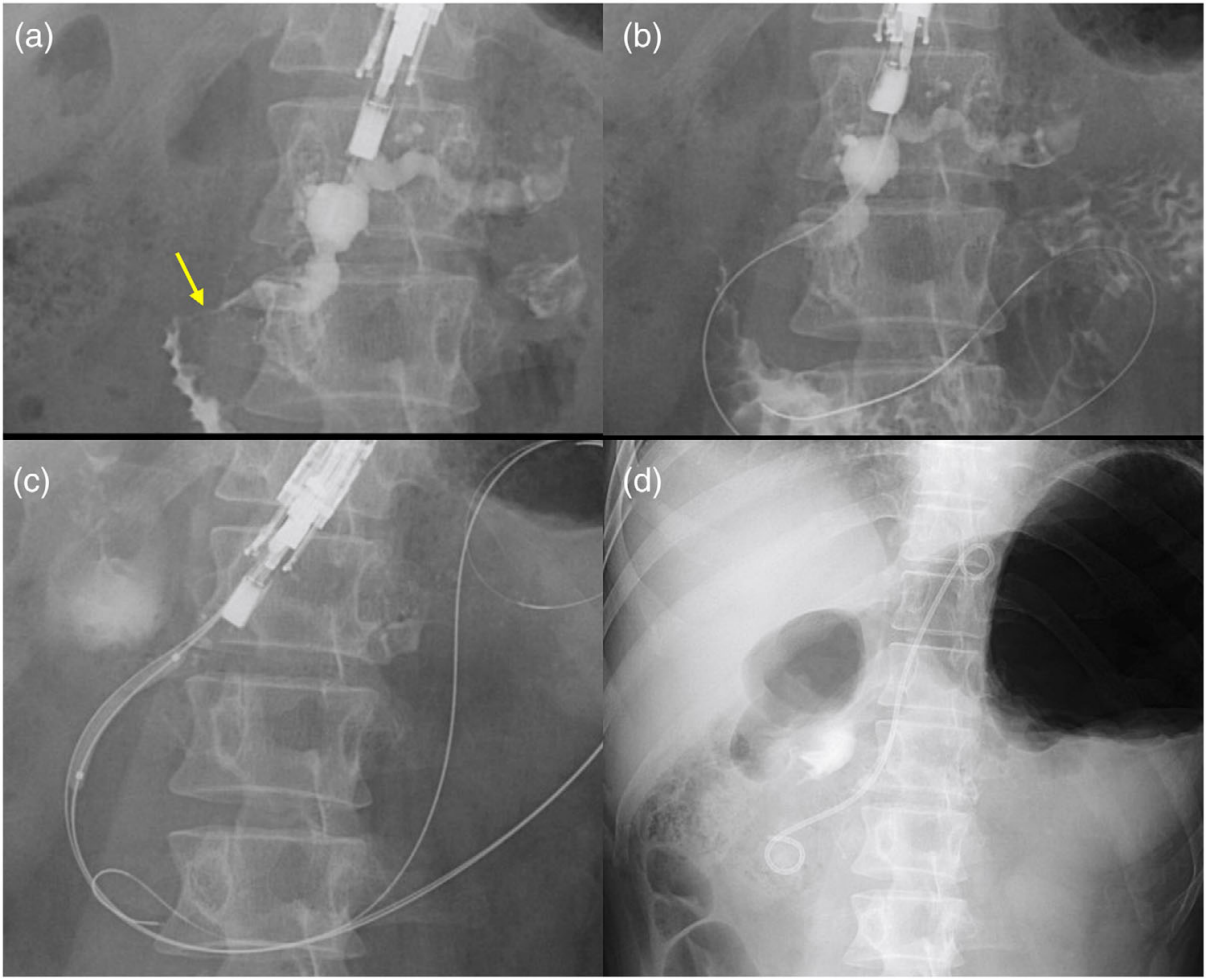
Endoscopic ultrasonography‐guided pancreaticogastrostomy: (a) The main pancreatic duct was punctured from the pancreatic body with a 19‐gauge needle (EZshot3; Olympus Medical Systems, Tokyo, Japan). Pancreatogram showed both the stenosis of Santorini's (arrow) and Wilsung's ducts. (b) A 0.025‐inch guidewire (Visiglide2; Olympus Medical Systems) was successfully passed through the Santorini's duct and minor papilla and placed in the duodenum. (c) Double guidewire technique with additional 0.035‐inch hard type guidewire placement (RevoWave, Ultrahard type; Piolax Medical Devices Inc., Kanagawa, Japan) was feasible and safe to dilate by balloon (REN; KANEKA Medical, Osaka, Japan). (d) A 7‐Fr x 15‐cm pigtail stent (Zimmon; Cook Medical, Bloomington, IN, USA) was placed through the Santorini's duct stenosis and minor papilla via the Endoscopic ultrasonography‐guided pancreaticogastrostomy route
